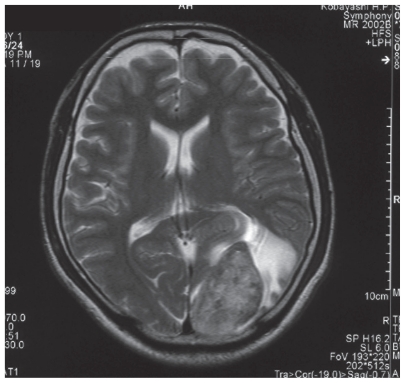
Brain MRI. The solitary metastatic lesion was shown, which was 4.5 cm × 3 cm in diameter, occupying the left occipital lobe (before operation).
